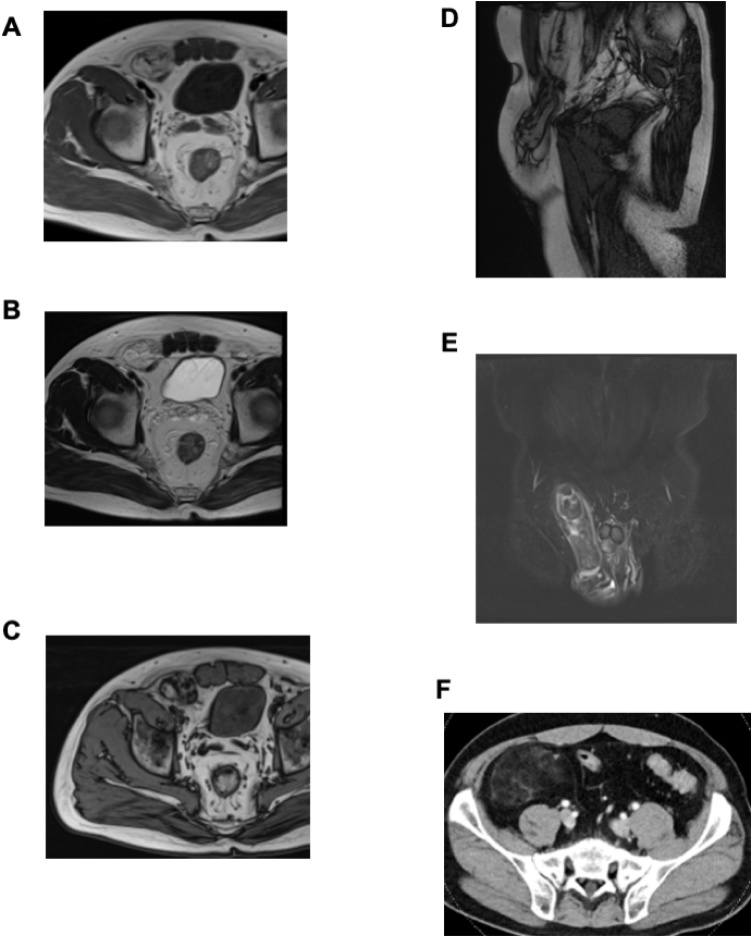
(A) Contrast-enhanced magnetic resonance imaging, showing partial solid components with low in T1 emphasized. (B) High in T2 emphasized. (C) Fatty components with high both in T1 and in T2 emphasized, however low in fat suppression images. (D) Sagittal image in T2. (E) Coronal image in T2 STIR. (F) Contrast-enhanced computed tomography revealed a tumor, including fatty tissue, measuring 80 × 60 mm in front of the major psoas muscle in the hypogastric region.
